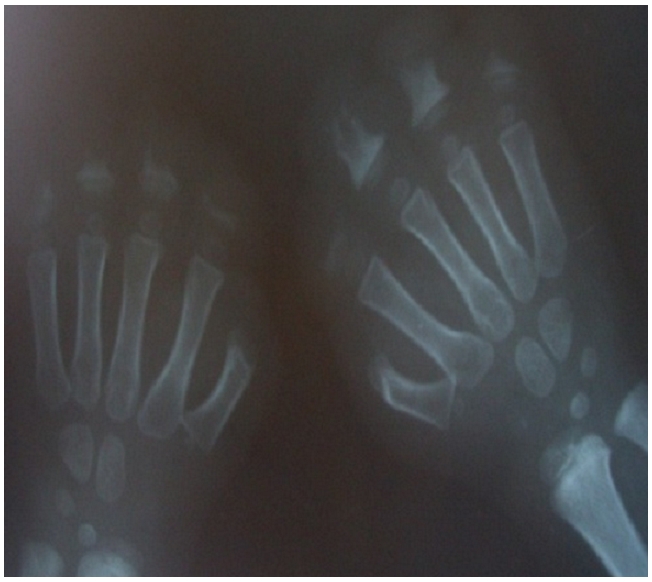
X-ray showing destruction and amputation of fingers in a child suffering from congenital insensitivity to pain with anhydrosis
